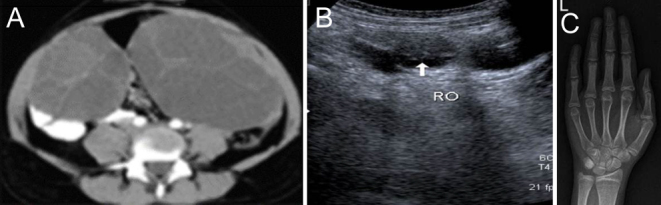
(A) CT abdomen at presentation with large multilocular cysts probable mucinous cystadenoma. (B) Ultrasound abdomen at follow-up with resolved cyst. (C) P2 presented at 16 years with delayed bone age of 9 years..
